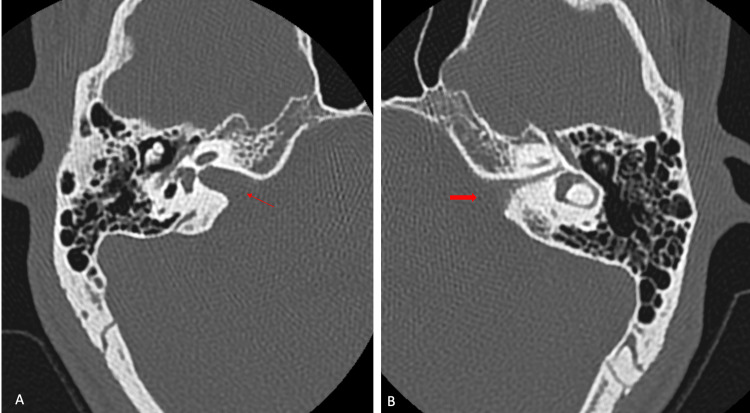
Axial planes of HRCT in bone window at level of IAC: (A) normal right IAC measuring 4.0 mm (thin red arrow) and (B) narrow left IAC measuring 1.4 mm (thick red arrow) HRCT: high-resolution CT, IAC: internal auditory canal
